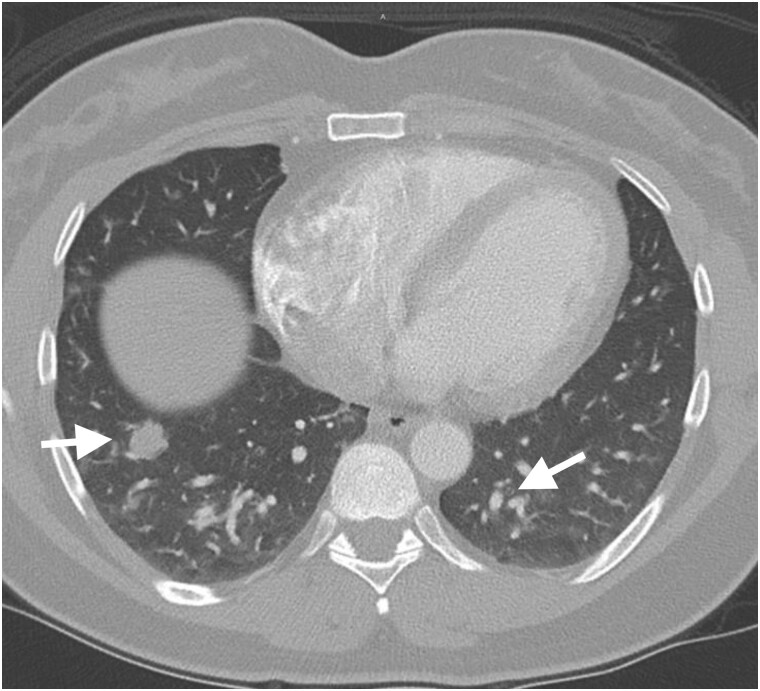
Contrast-enhanced chest computed tomography in lung window demonstrates multiple pulmonary nodules and mass lesions in the lungs bilaterally. Radiographic differential considerations would include both neoplastic and infectious etiologies.
